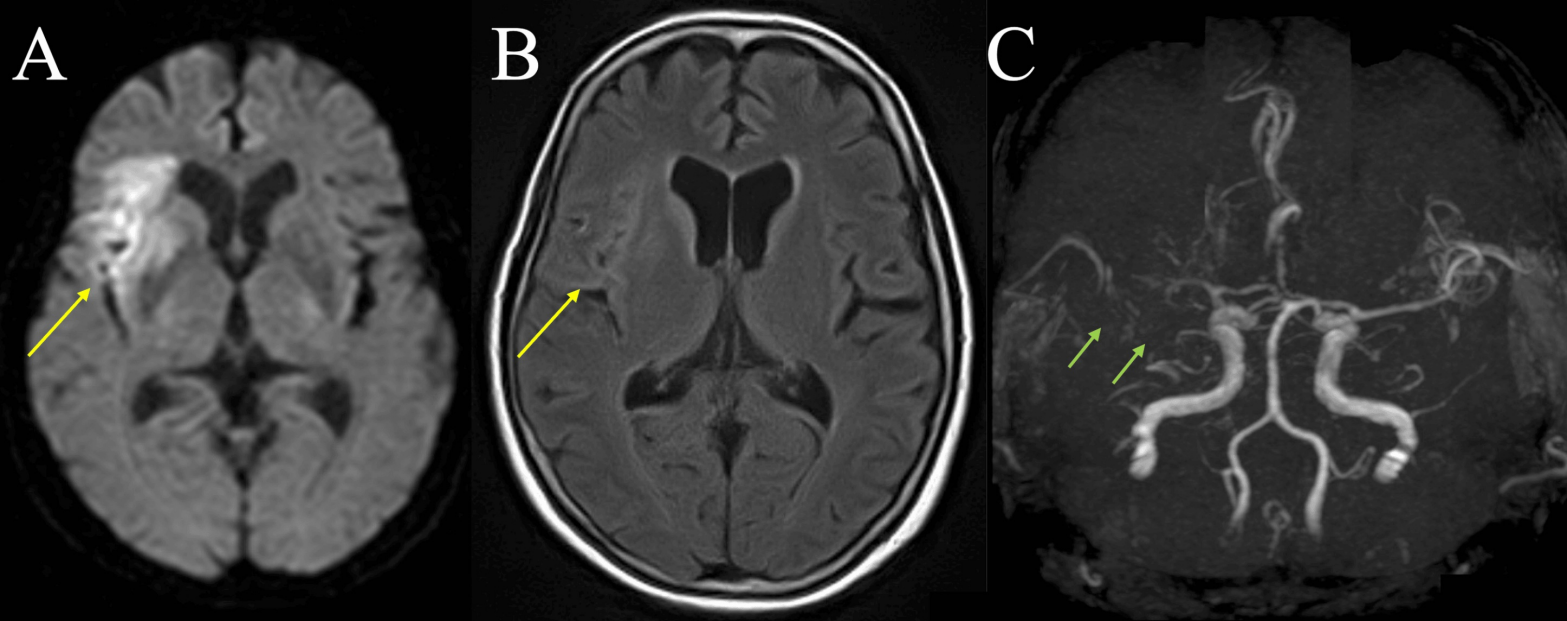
Brain MRI and MRA findings demonstrating multiple embolic strokes (A-C) Brain MRI on hospital day 50. (A) Diffusion-weighted imaging showing a hyper signal intense lesion in the right basal ganglia (yellow arrow). (B) Fluid-attenuated inversion recovery image showing a lesion in the same region. (C) MRA showing the occlusion of the right middle cerebral artery (green arrow). MRI: magnetic resonance imaging; MRA: magnetic resonance angiography
